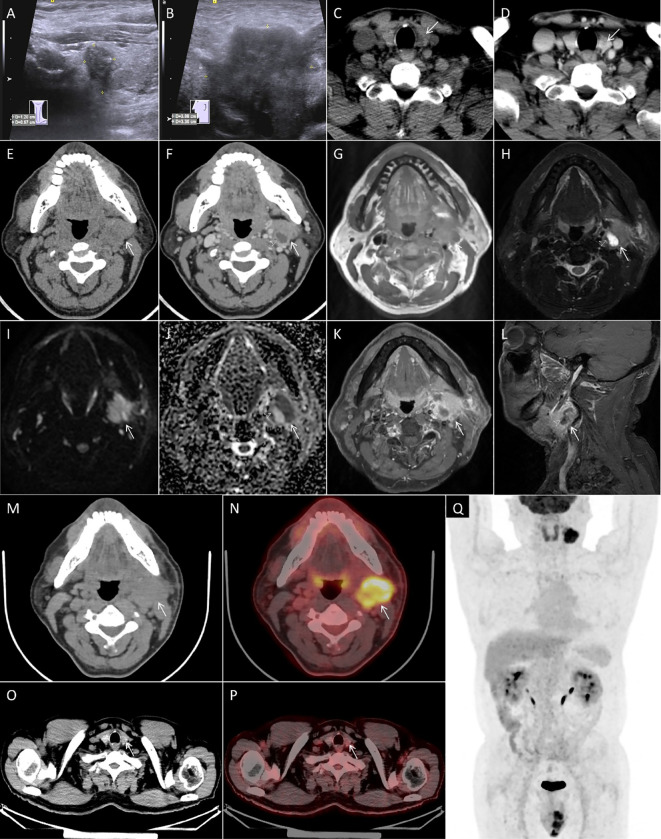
Results of the patient’s neck US, CT, MRI, and ^18^F-FDG PET/CT scans. US showed a 1.2 × 1.0cm hypoechoic nodule in the left thyroid upper pole (A), classified as 4C according to the Chinese Thyroid Imaging Reporting and Data System (C-TIRADS) (6). US revealed a 3.3 × 3.1cm hypoechoic lesion in the left submandibular region (B). CT demonstrated a suspected hypodense nodule in the left thyroid upper pole (white arrow in (C, D)). CT and MRI revealed an irregular left submandibular region mass (≈3.6 × 2.9 × 3.6cm), suspicious for malignancy (white arrow in (E–L)). PET/CT revealed intense uptake in the left submandibular region mass, with a maximum standardized uptake value (SUV_max_) of 8.0 (white arrow in (M, N)), and mild uptake in the thyroid nodule (SUV_max_ = 1.4) (white arrow in (O, P)), with no other foci of significantly increased uptake (Q).
